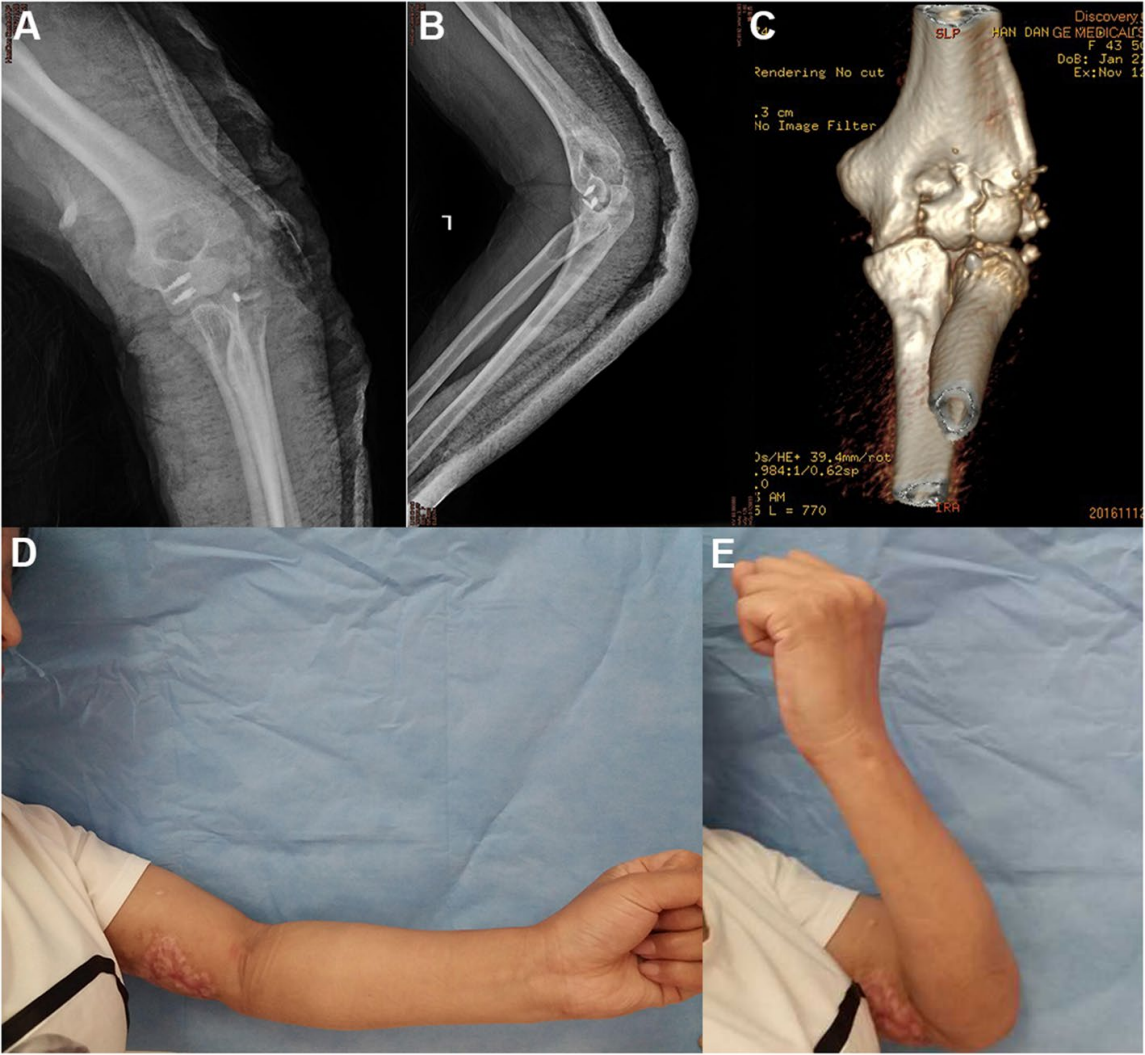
Case 2: A postoperative X-ray (A and B) and spiral CT scan (C) reveal that the radial head and humeral capitulum fractures are in good alignment, and the reduction of the brachial radial joint is satisfactory. The patient is followed up at 10 months after the operation, at which time the extension and flexion functions of the left elbow joint are satisfactory (D and E)
